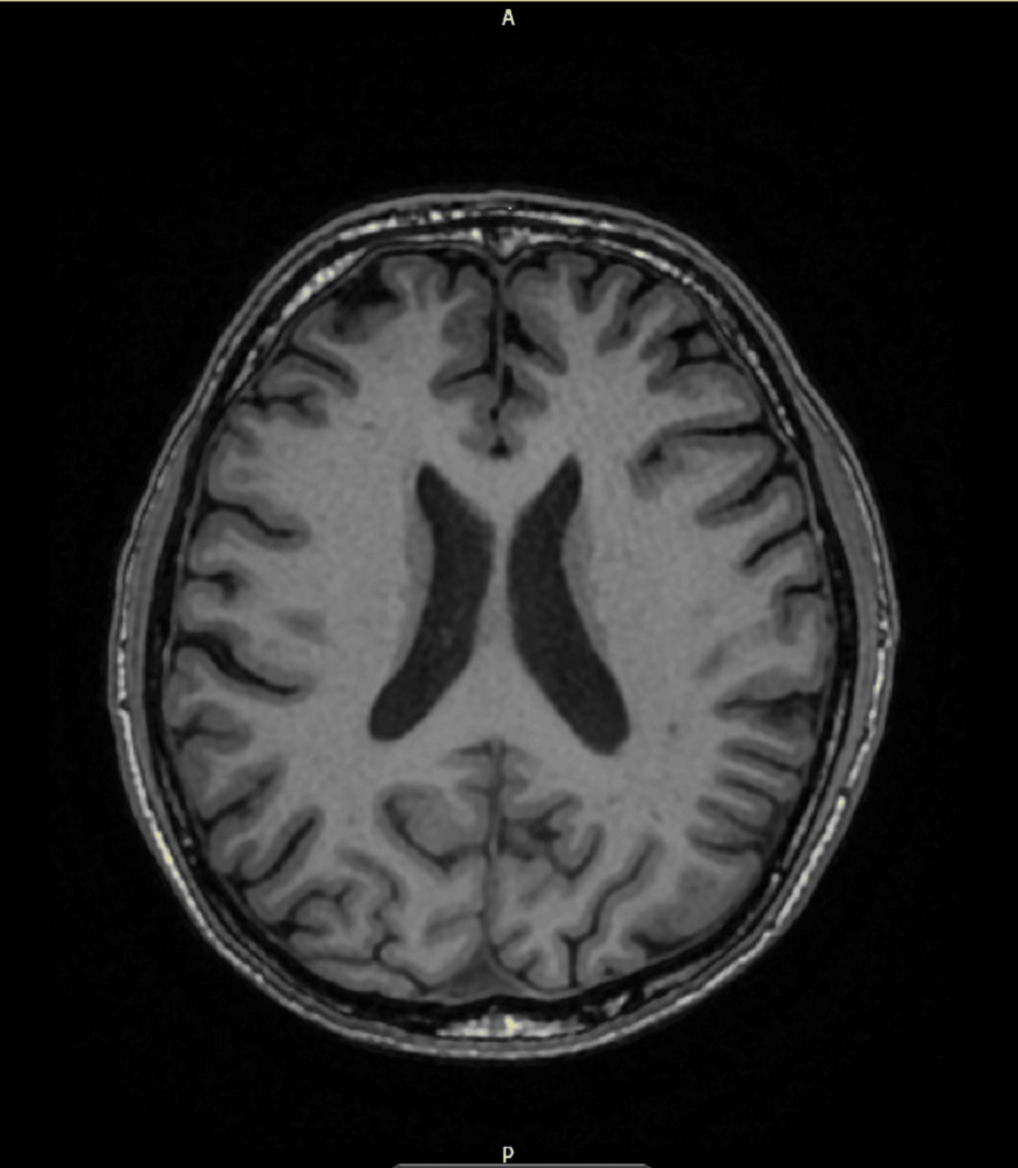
Normal MRI brain There’s no sign of intracranial pathology or demyelinating disorder with normal CSF appearance. Therefore, the MRI is basically unremarkable. Excluding potential differentials. CSF: cerebrospinal fluid, MRI: magnetic resonance imaging
